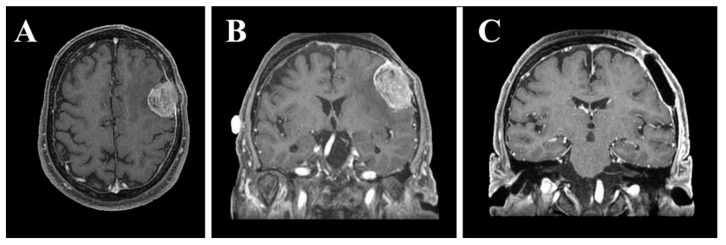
Preoperative axial (A) and coronal (B) gadolinium-enhanced T1-weighted MRI showing en plaque convexity meningioma. Postoperative coronal gadolinium-enhanced T1-weighted MRI (C) showing surgical resection of the tumor and cranioplasty with polymethylmethacrylate.
